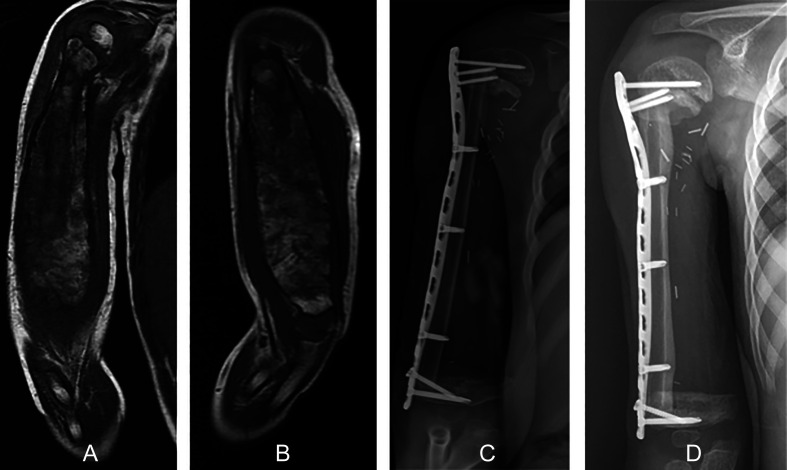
A six-year-old boy, diagnosed with Ewing sarcoma, who was treated by intercalary resection followed by reconstruction of the right humerus using a free-vascularized fibular graft. Coronal (A) and sagittal (B) T1 magnetic resonance images show a tumor located at the diaphyseal region of the humerus without involvement of the proximal or distal growth plates. A postoperative anteroposterior radiographic view (C) shows an intercalary reconstruction of the humerus with a free-vascularized fibular graft. (D) Radiograph showing initial consolidation of the fibular graft at the two-month follow-up. Although fixation was possible with just a few screws, the fibula graft was able to integrate within two months, making the reconstruction stable.
